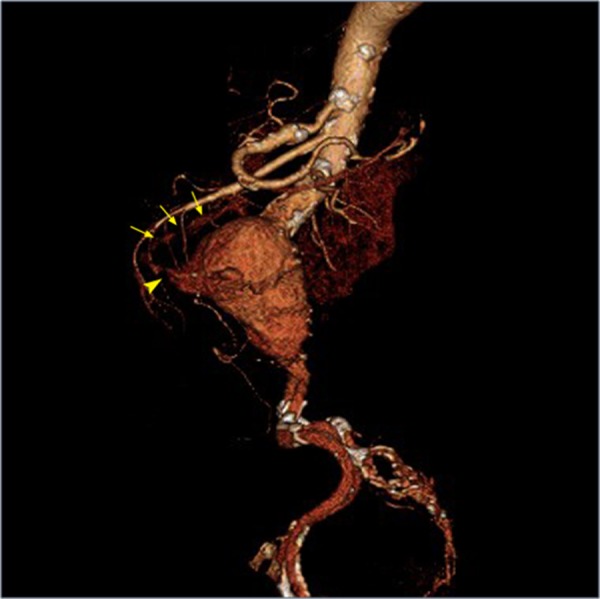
3D Reconstruction of PAEF Left lateral 3D reconstructed contrast-enhanced (IV only) CT image showing the presence of an AAA, fistula (arrowhead), and layering contrast within the 3rd part of the duodenum (arrows).
